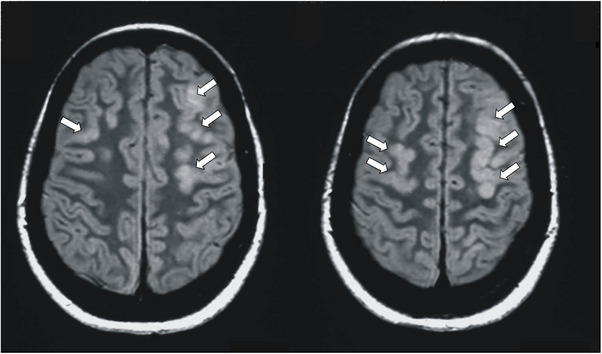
Brain MRI showing multiple, bilateral, and predominantly frontal and parietal, cortical–subcortical lesions, hypointense on T1‐weighted images and hyperintense on T2/FLAIR sequences.
